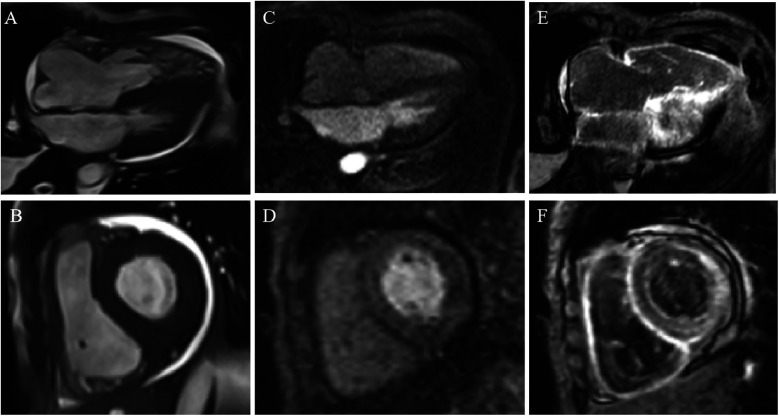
Cardiac magnetic resonance images. (A,B) The images of cine scan. (C,D) The images of first-pass myocardial perfusion scan. (E,F) Late gadolinium enhancement (LGE) imaging showed diffuse LGE.
